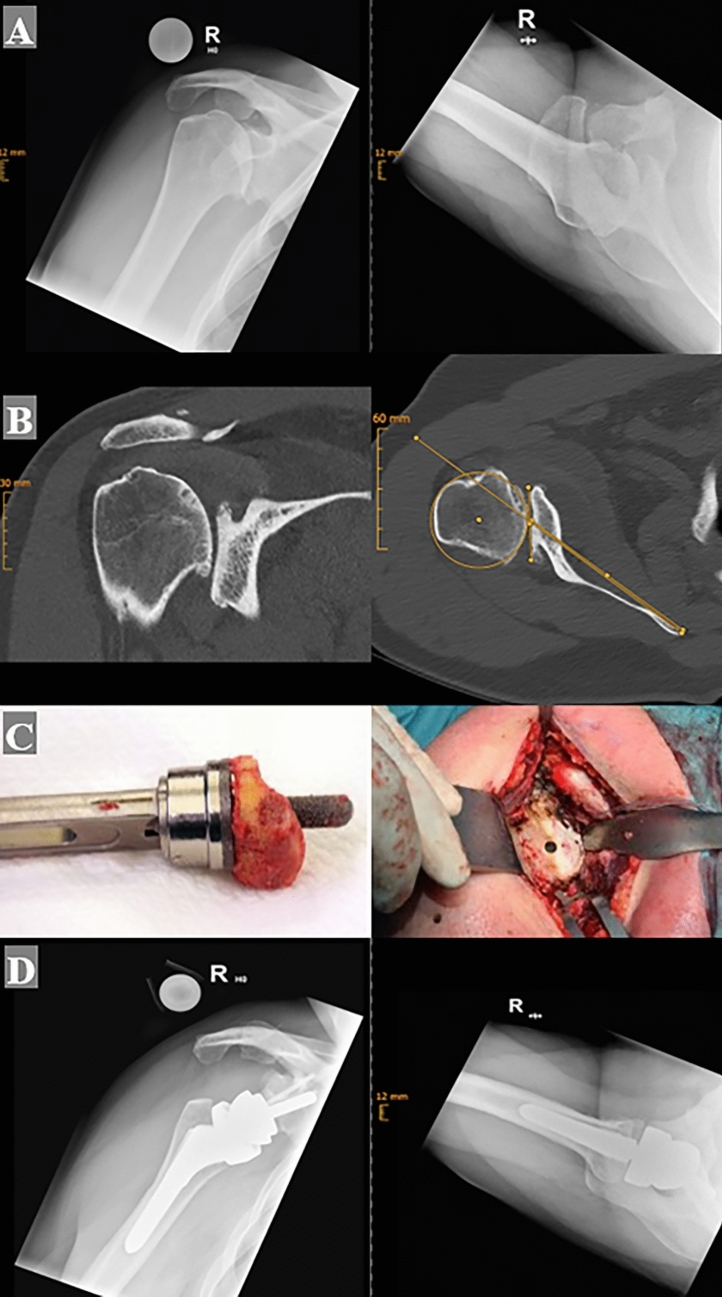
Fifty-seven-year-old man with primary glenohumeral osteoarthritis and a posterior bony deficiency (Walch type C). Preoperative radiograph and computed tomography–scan (a and b), intraoperative reconstruction of the glenoid using of a structural humeral head autograft (c). Post-operative radiograph (d)
